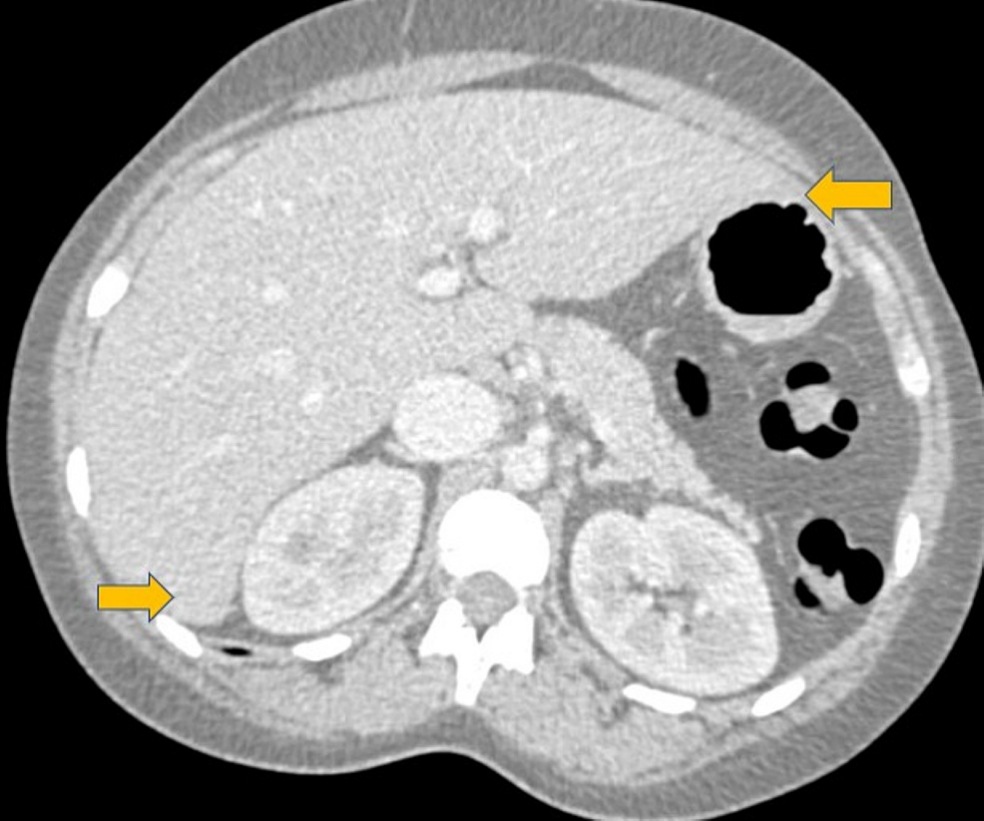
Computed tomography of abdomen Computed tomography of abdomen and pelvis with intravenous contrast demonstrating hepatomegaly with a span of 25 cm (yellow arrows). When compared to prior imaging studies obtained 10 months prior, the patient’s hepatomegaly was a new and significant change.
